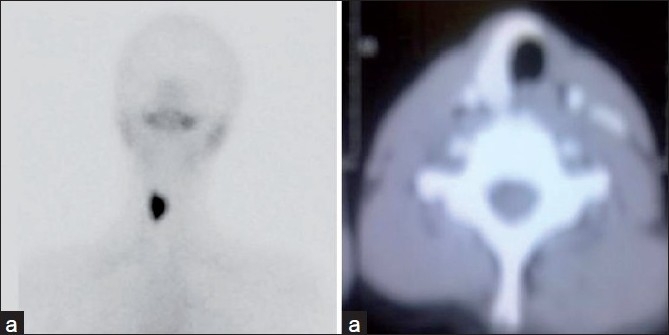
(a, b) Thyroid scan and CT image (axial section) showing thyroid hemiagenesis
